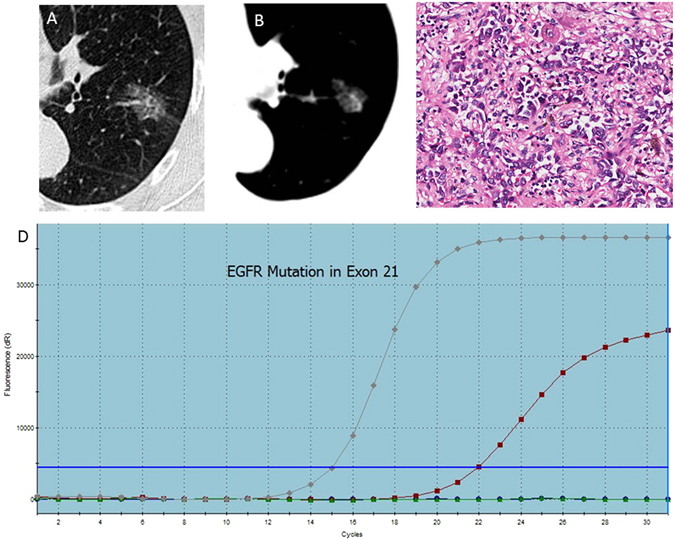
A non-smoking woman with lung adenocarcinoma in the left upper lobe, where PCR images (A) and pathological picture (D) show that the subtype is exon 21 mutation. CT images (B,C) show a subsolid lump with spiculated margins.
